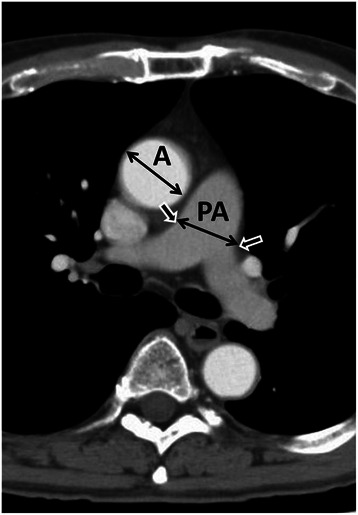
Measurement of the diameters of the pulmonary artery and aorta. Axial chest computed tomographic image at the level of the bifurcation of the main pulmonary artery. Measurements of the diameter of the main pulmonary artery (PA) and that of the aorta (A) at the level of the bifurcation were used to calculate the PA/A ratio. Short arrows show the rises of the bilateral pulmonary arteries. It is the measuring point of the diameter of PA
